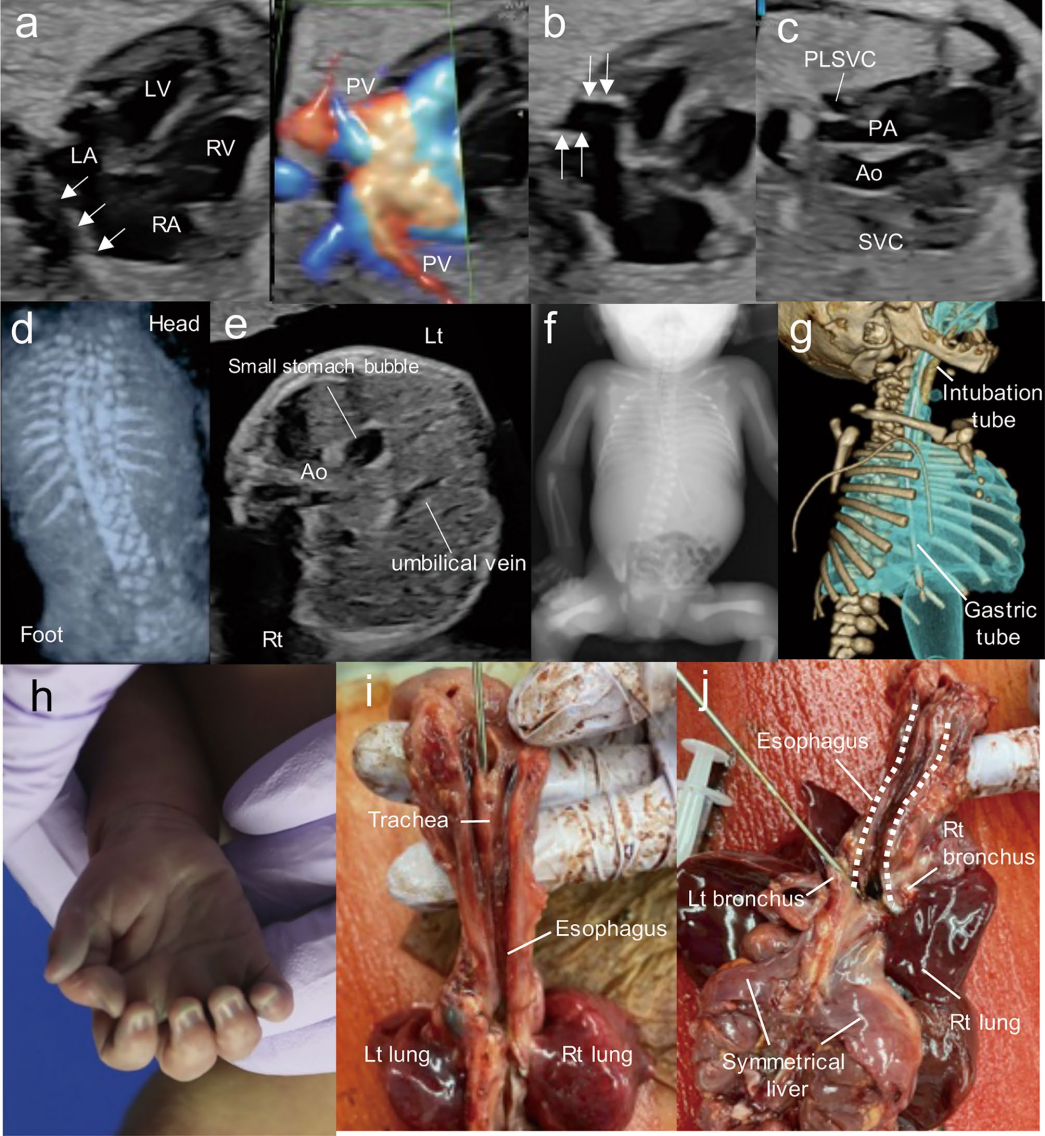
Clinical features of a case with a *RAC1* variant. (a–e) Fetal ultrasonography of the proband at 28 weeks of gestation. (a) Posterior venous confluence (white arrows) was recognized on a 4-chamber view of isolated total anomalous pulmonary venous return (TAPVR). (b) Enlarged coronary sinus (white arrows) implied persistent left superior vena cava (PLSVC). (c) Abnormal three vessel view showed the PLSVC. (d) Fetal spine 3D ultrasonography with skeleton mode indicated scoliosis. (e) Small stomach bubble implied esophageal atresia on axial view, which was a finding associated with tracheal agenesis type III. (f) Scoliosis was recognized at birth by X-ray imaging. (g) 3D computed tomography imaging showed that an intubation tube was not located at the bifurcation of the trachea, indicating tracheal agenesis type III. (h–j) Photographs of the patient at autopsy. He had right-hand polydactyly (h). The trachea was blind-ended (i) and the esophagus was connected to the right lung, indicating tracheal agenesis type III and esophagotracheal fistula (j), respectively. Symmetrical liver was present (j). *Ao* aorta, *LA* left atrium, *Lt* left, *LV* left ventricle, *RA* right atrium, *Rt* right, *RV* right ventricle, *PV* pulmonary vein, *PLSVC* persistent left superior vena cava.
